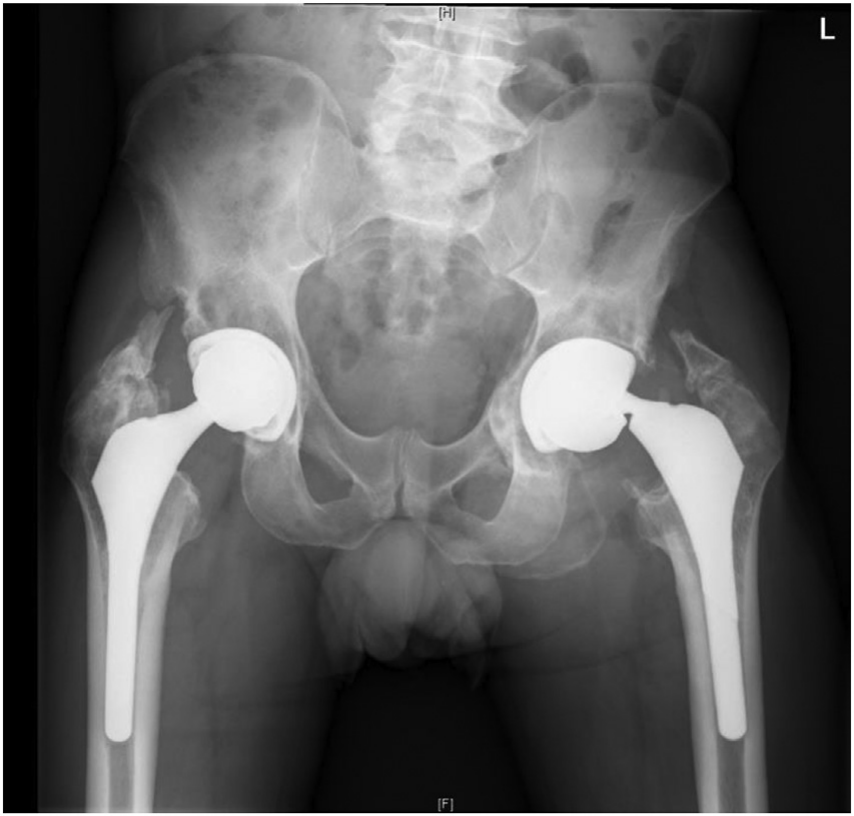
Anteroposterior pelvis radiograph of Case 1. A 62-year-old man with bilateral metal-on-metal total hip arthroplasties (THAs) and impending catastrophic failure of the left THA.
